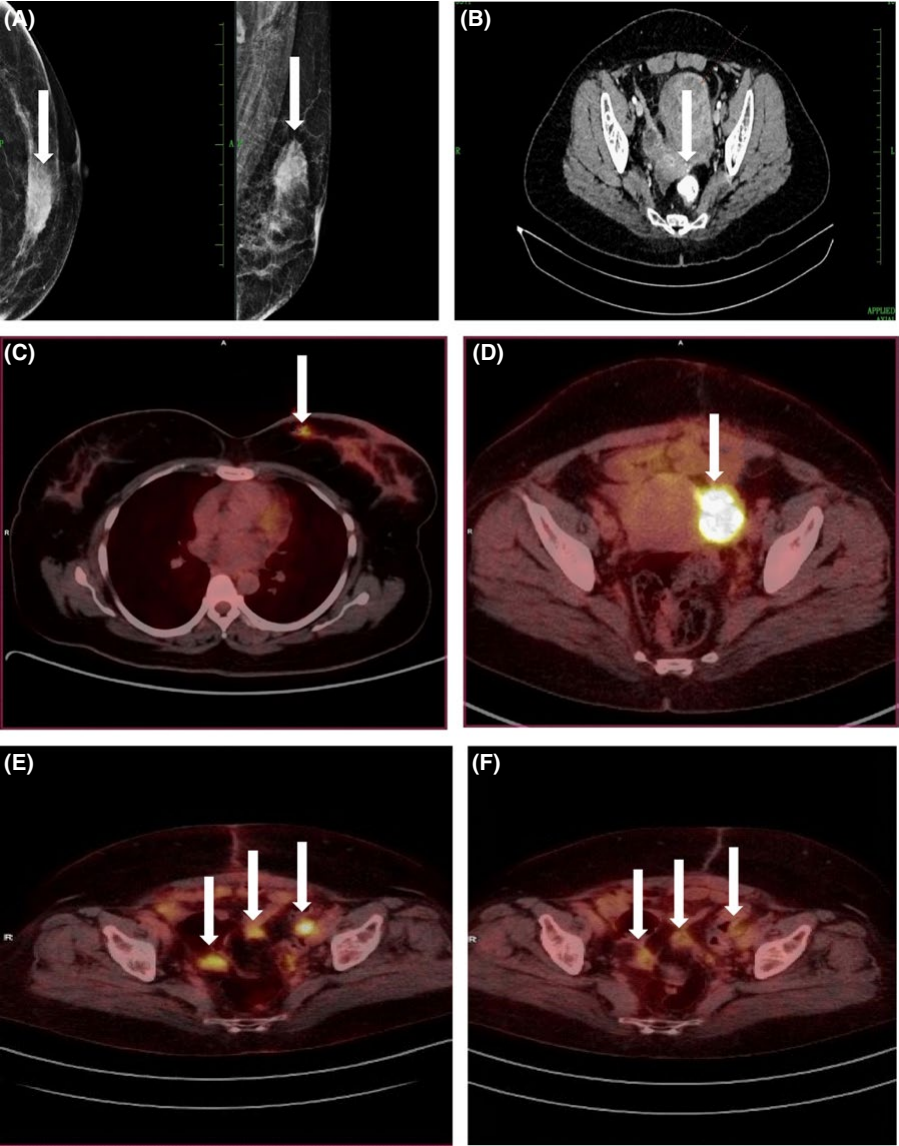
Screening of breast and ovarian tumors by imaging. (A) Left image: mammogram of left breast lesion indicated by an arrow. (B) CT scan of ovarian lesion indicated by an arrow. (C and D) FDG‐PET/CT image of breast and ovarian lesions indicated by an arrow, respectively. (E) FDG‐PET/CT image of ovarian tumor before neoadjuvant paclitaxel and carboplatin drug therapy, and (F) tumor size reduction after three cycles of combined drug therapy with lesions indicated by arrows before and after therapy
